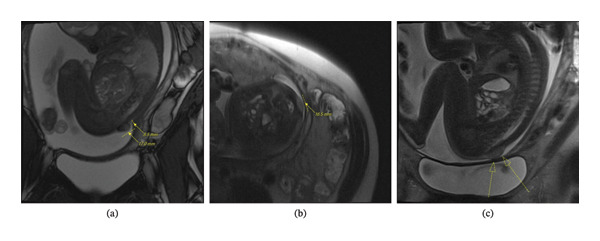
MRI of the fetus in utero. (a) Sagittal. (b). Transverse. (c) Sagittal. MRI findings demonstrated a 17 × 16.5 × 8.5 mm discoid homogenous T2 mildly hyperintense soft tissue mass at the base of the spine.
